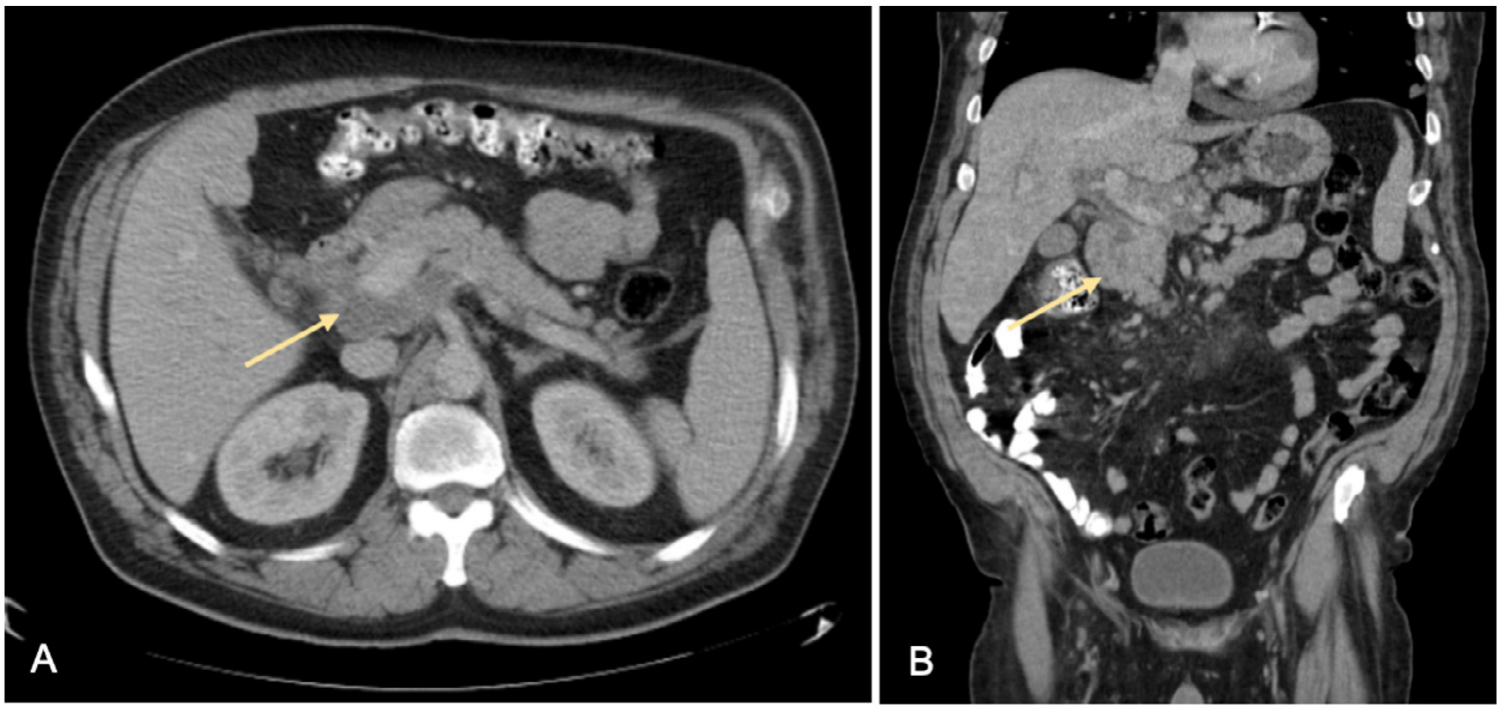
Axial (A) and coronal (B) images from a CT chest abdomen and pelvis with contrast showing a hypodense mass by the uncinate process of the pancreas measuring 5.6 by 2.7 cm.
